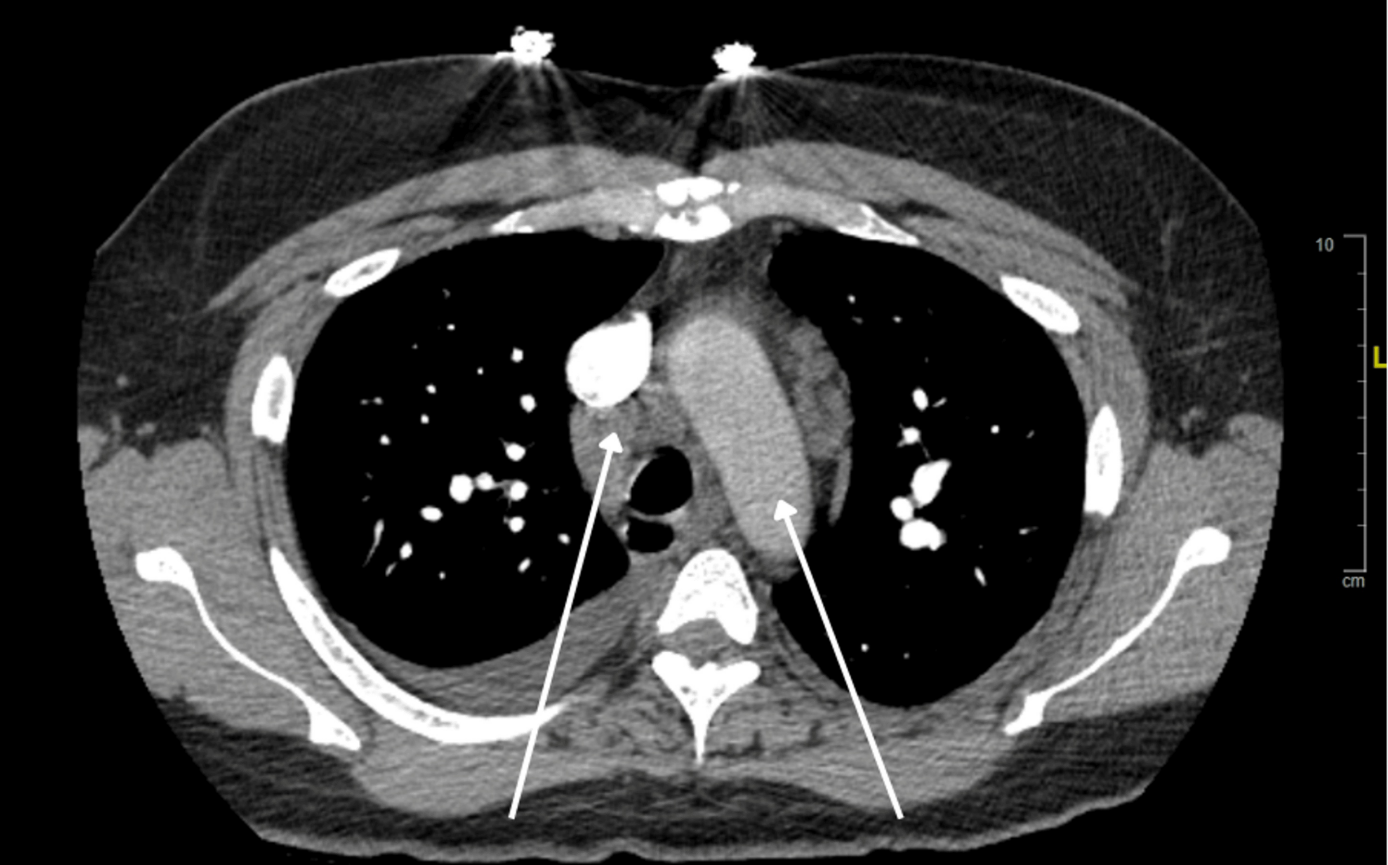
CT chest with contrast without evidence of pulmonary embolism Findings labelled by white arrow
